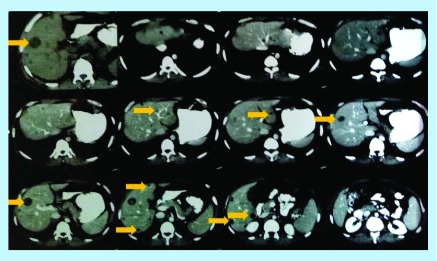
CT scan performed 30 months after surgery for primary leiomyosarcomas showing multiple hypodense lesions in the liver.
